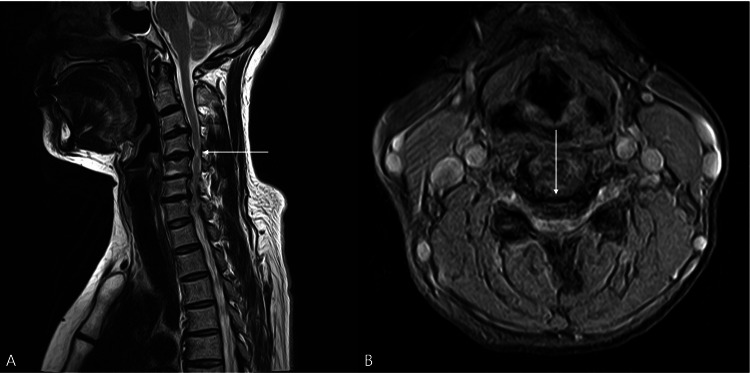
Sagittal and axial T2-weighted magnetic resonance imaging of the cervical spine. (A) Sagittal T2-weighted image demonstrates multilevel disc protrusion from C4 to C7 with associated spinal cord compression. Increased intramedullary signal intensity at the C4 level is observed, consistent with myelopathic change (white arrow). (B) Axial T2-weighted image at the C4 disc level, corresponding to the site of intramedullary signal change, demonstrates focal central canal narrowing with anterior disc protrusion causing direct ventral compression of the spinal cord (white arrow).
